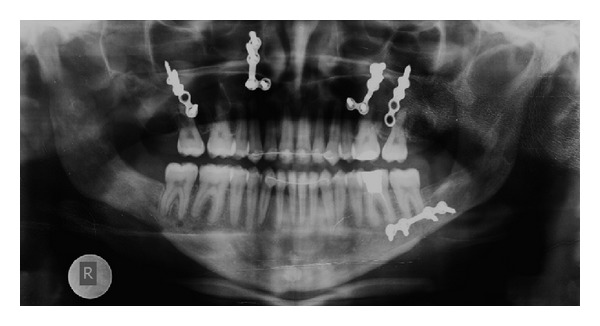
Posttreatment orthopantomogram (OPG).
